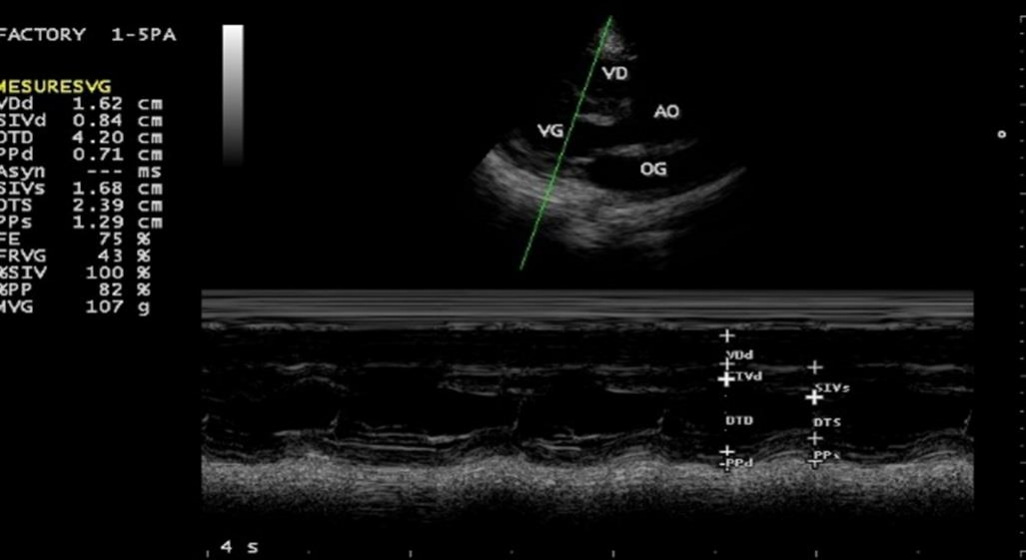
Post-opératoire (3 mois): coupe parasternale grand axe, mode bidimensionnel (image en haut) et mode TM (image en bas), VD de taille normale; VG de taille normale avec une bonne fonction systolique
